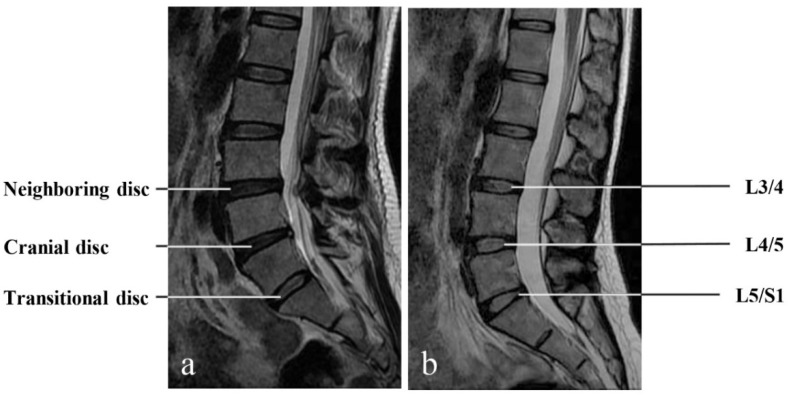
The most caudal three intervertebral discs were evaluated on T2W sagittal MR images. In the presence of LSTV, they were defined as the transitional disc, the cranial disc, and the neighboring disc (a), which corresponded to L5/S1, L4/5, and L3/4 in controls without LSTV, respectively (b). Spinal level-specific comparisons were performed for various measurements between LSTV patients and controls.
